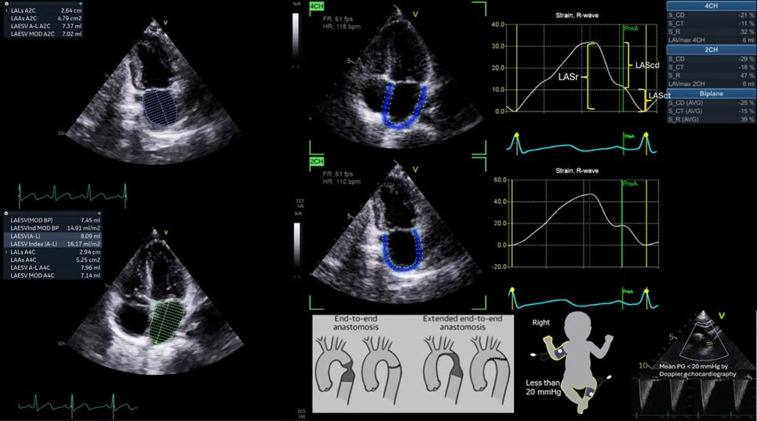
Representative left atrial phasic strain analysis by speckle-tracking echocardiography in a child post-coarctation repair. Apical four-chamber (top) and two-chamber (bottom) views demonstrate LA endocardial tracking and corresponding strain curves aligned to the R-wave. Phasic strain components: reservoir (S-R), conduit (S-CD), and contraction (S-CT) were derived using biplane assessment.
